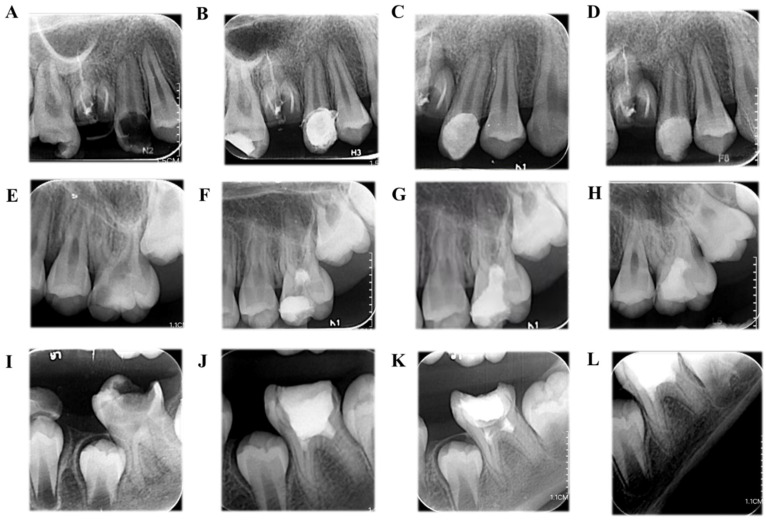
(A) The preoperative periapical radiograph of the upper-right second premolar in an 18-year-old male patient with irreversible pulpitis, clinical symptoms and mature roots. (B) The postoperative periapical radiograph after direct pulp-capping (DPC) with iRoot BP Plus. (C) The 12-month follow-up. (D) The 24-month follow-up (E) The preoperative periapical radiograph of the upper left first molar in a 13-year-old male patient with irreversible pulpitis of clinical symptoms and mature roots. (F) The postoperative periapical radiograph after partial pulpotomy (PP) with iRoot BP Plus. (G) The 6-month follow-up. (H) The 12-month follow-up. (I) The preoperative periapical radiograph of the lower right first molar in a 9-year-old female patient with irreversible pulpitis of clinical symptoms and immature roots. (J) The postoperative periapical radiograph after full pulpotomy (FP) with iRoot BP Plus. (K) The 12-month follow-up. (L) The 24-month follow-up showing continued root development.
